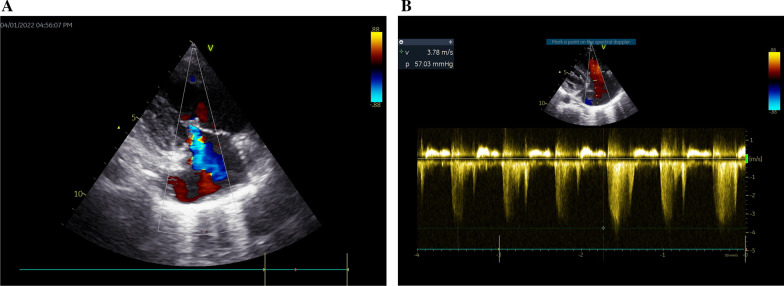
A Tricuspid regurgitant jet. B Continuous wave Doppler gradient across the across the tricuspid regurgitant jet
